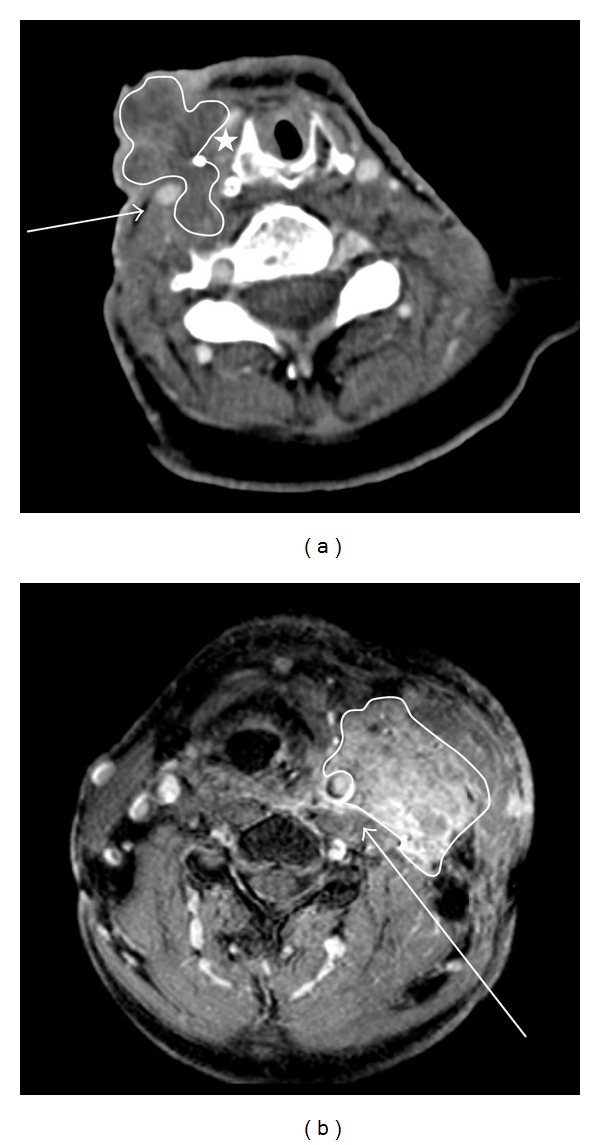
Examples of CT and MR images showing carotid encasement. (a) Axial CT image of a lymph node metastases (the mass is encircled by a white line) at the right side showing at least 270 degrees of encasement. The confluent lymph node mass is invading into the skin. The right carotid artery (arrow) is covered by the lymph node mass. Note: the right internal jugular vein is not visible, possibly due to compression. Suggestive the high-density structure (white star) lateral to the right lamina of the cricoid is surgical clip from earlier operation. (b) Fat-suppressed T1 contrast-enhanced MR section showing lymph node metastases in the left neck. The left internal carotid artery (arrow) is covered anteriorly and laterally by nodal disease (the mass is encircled by a white line). The circumferential involvement is (just) over 180 degrees.
